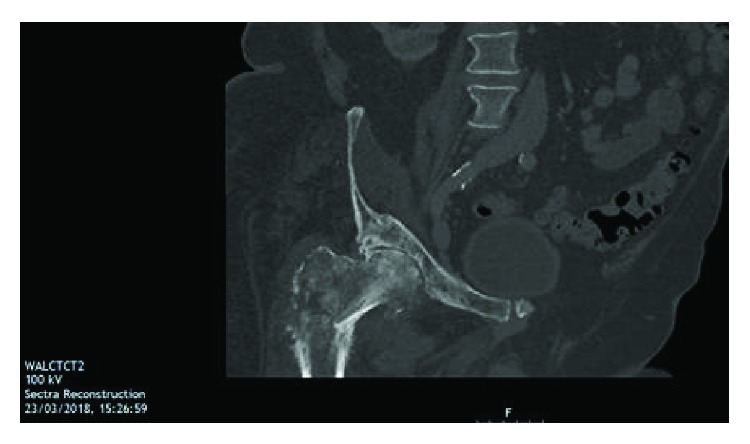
Computed tomography, coronal plane, demonstrating a bony lesion affecting the right acetabulum and proximal femur with associated proximal femoral fracture.
